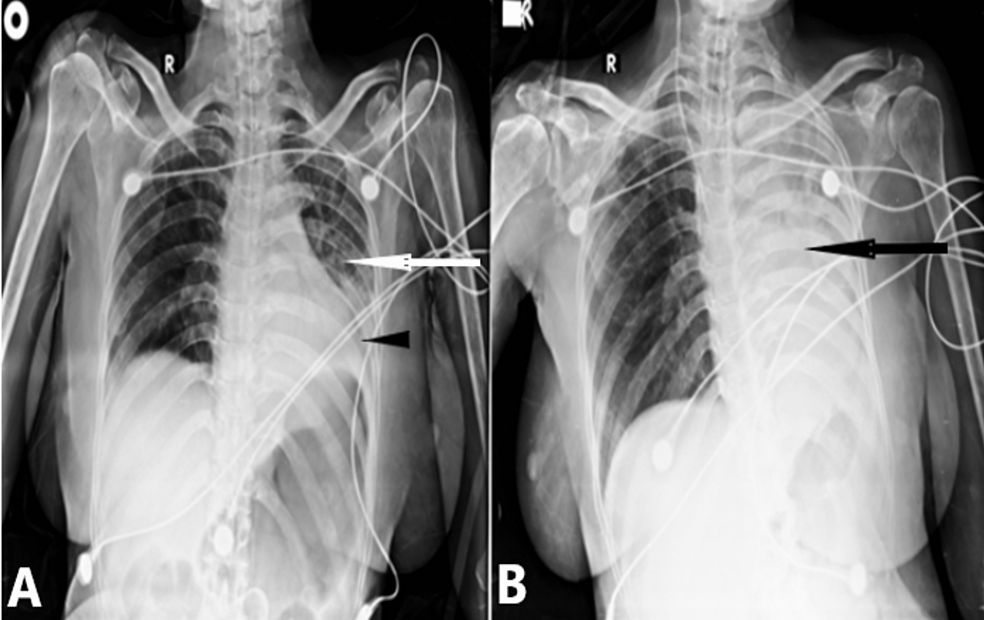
Case 4 A 45-year-old COVID-19-positive patient came with complaints of fever and cough for six days with on-admission oxygen saturation of 60% on room air. She underwent invasive ventilation. (A) On admission, a chest radiograph shows airspace opacities in the left upper and middle lung zones pointed by a white arrow suggestive of patchy consolidatory changes with mild left-sided pleural effusion shown by a black arrowhead. (B) Follow-up X-ray after five days showed a white-out left lung parenchyma indicated by a black arrow suggestive of massive pleural effusion. The patient succumbed to death three days later because of respiratory failure.
